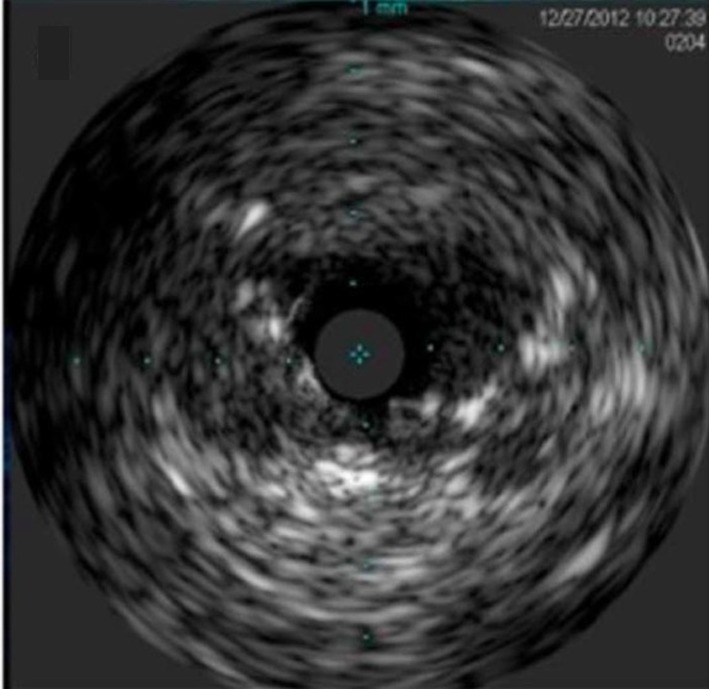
Intra-vascular ultrasound (IVUS) dynamic flap outside the left main ostium.
